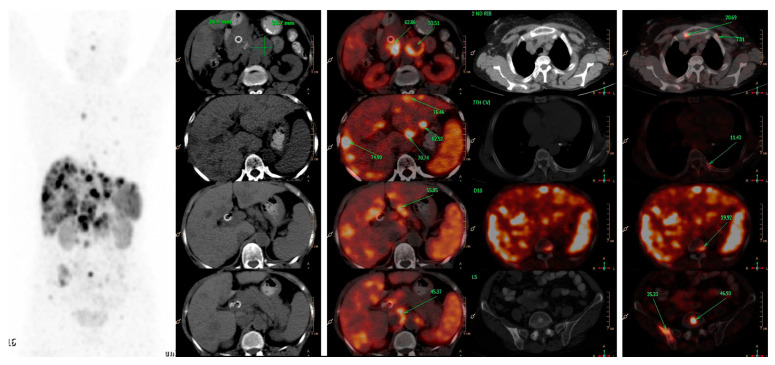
A 66-years-old female patient, presented with features of obstructive jaundice. Triphasic CECT scan abdomen showed large hypodense SOL in head of pancreas encasing CBD with significant upstream dilatation of biliary tree with multiple hypodense bilobar hepatic metastases and multiple abdominal & retroperitoneal lymph nodes. Patient was referred for PRRT and ^68^Ga-DOTATATE PET-CT was done which confirmed above-mentioned triphasic CE-CT scan findings and showed many new skeletal and marrow lesions at multiple skeletal sites. Hence is SSTR-based PET-CT represents a better modality for metastatic workup than conventional imaging.
